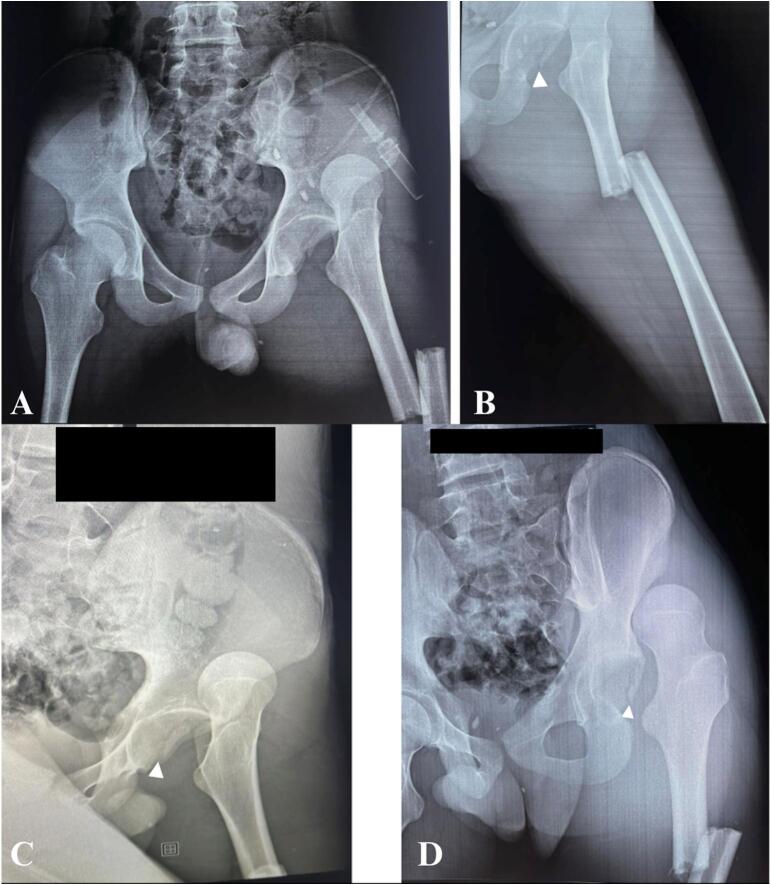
A + B + C + D: Radiographs demonstrating a left hip fracture dislocation with an acetabular posterior wall fracture (white arrows) associated with left femoral and shaft fractures (C: Iliac oblique view, D: Obturator oblique view).
